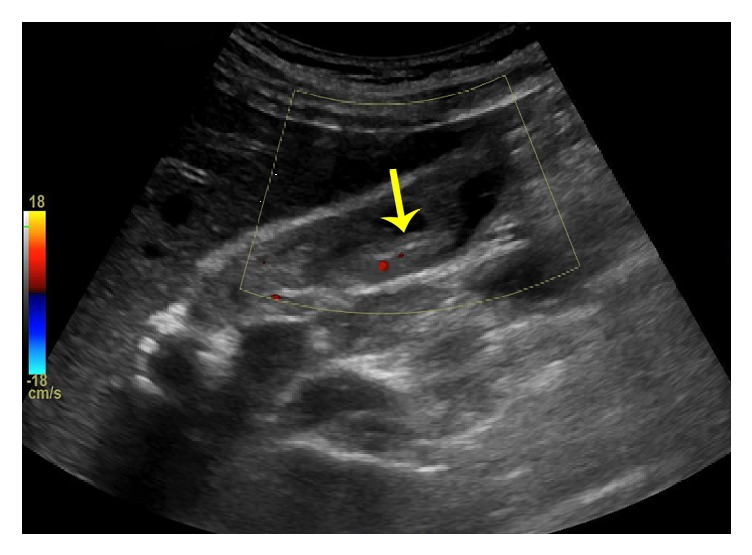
Abdominal ultrasound with filling defect in bile duct.
